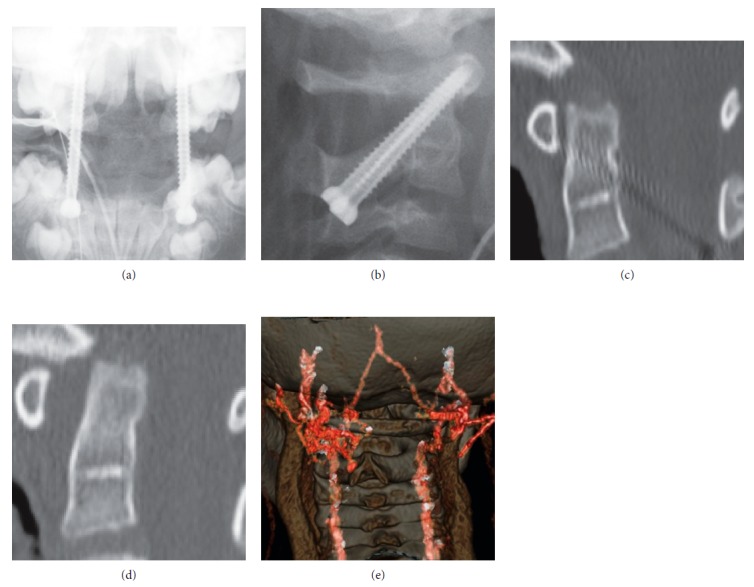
Result of C1-C2 Magerl surgery without a bone graft (a, b). Odontoid process shows osteolysis after C1-C2 Magerl surgery (c). Type 2 AARF recurred 2 weeks after the screw removal. The reconstruction CT shows osteolysis of the odontoid process (d). Preoperative 3D CT showed hypervascularity posterior to C1 (e).
